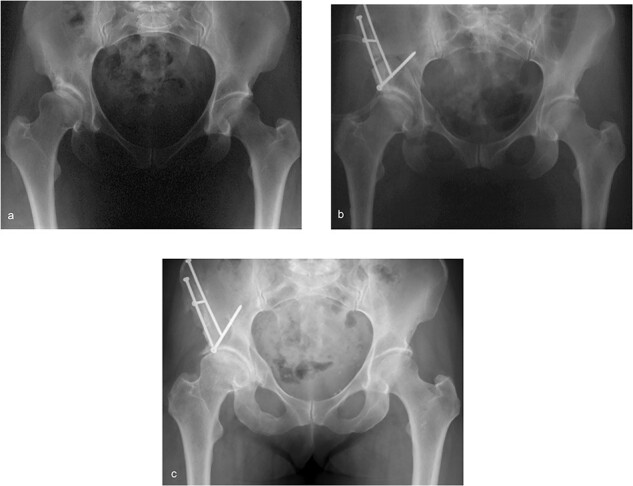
(a) Preoperative, (b) postoperative and (c) 10-year follow-up AP pelvis radiographs of a female patient with DDH who underwent periacetabular reorientation osteotomy.
